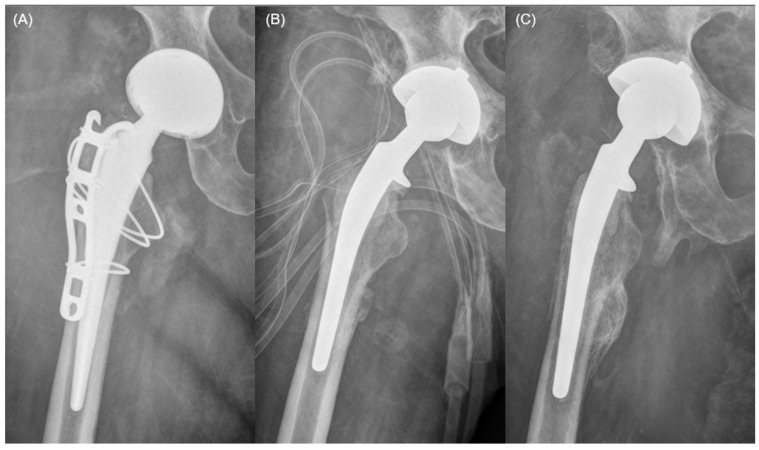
Serial radiographs of a patient who retained the dual-mobility bearing (DMB)-incorporated functional articulating hip spacer (FAHS) over long-term follow-up. (A) A 60-year-old woman developed PJI following primary total hip arthroplasty (THA) performed at an outside hospital. (B) After removal of all implants, a DMB-incorporated FAHS was implanted. (C) At 39.2 months postoperatively, the patient remained infection-free and continued to retain the DMB-incorporated FAHS without undergoing second-stage THA, per patient preference.
